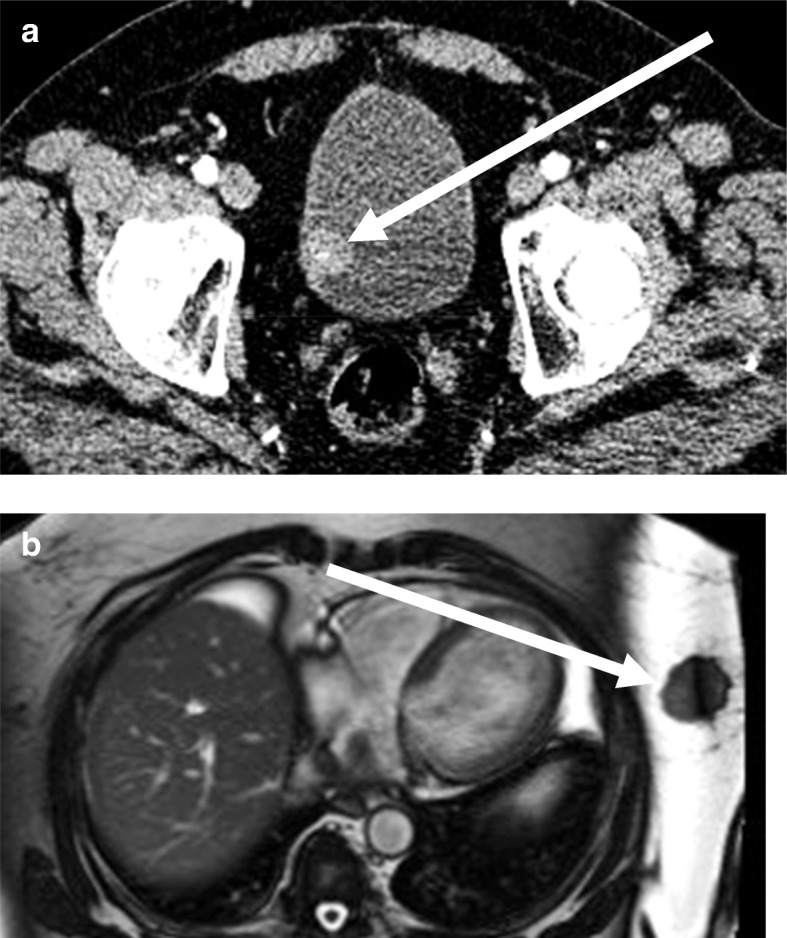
a Missed incidental cancer on CT (case 13, Nov 2012). b ‘Good spot’ incidental cancer on MR
